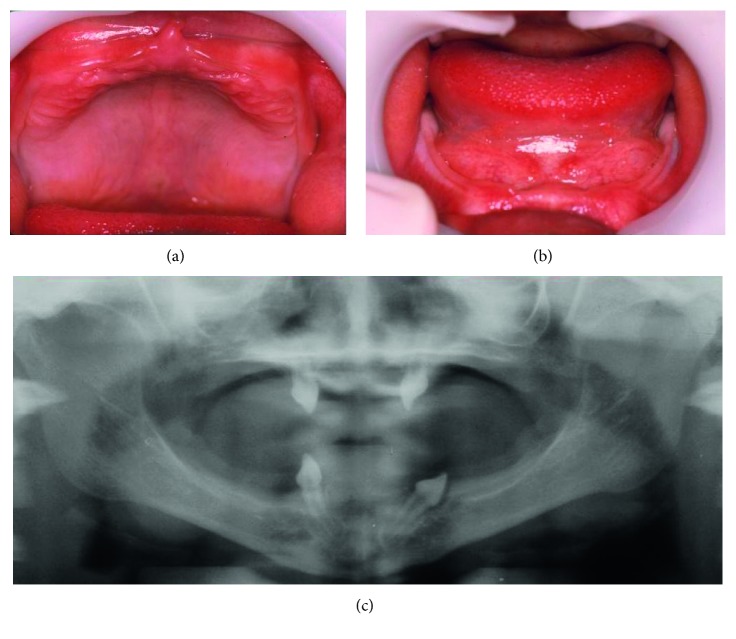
Patient 3. (a) Upper and (b) lower alveolar ridges were edentulous and underdeveloped, and (c) radiographic images showing the presence of the 53rd, 63rd, 73rd, and 83rd teeth.
